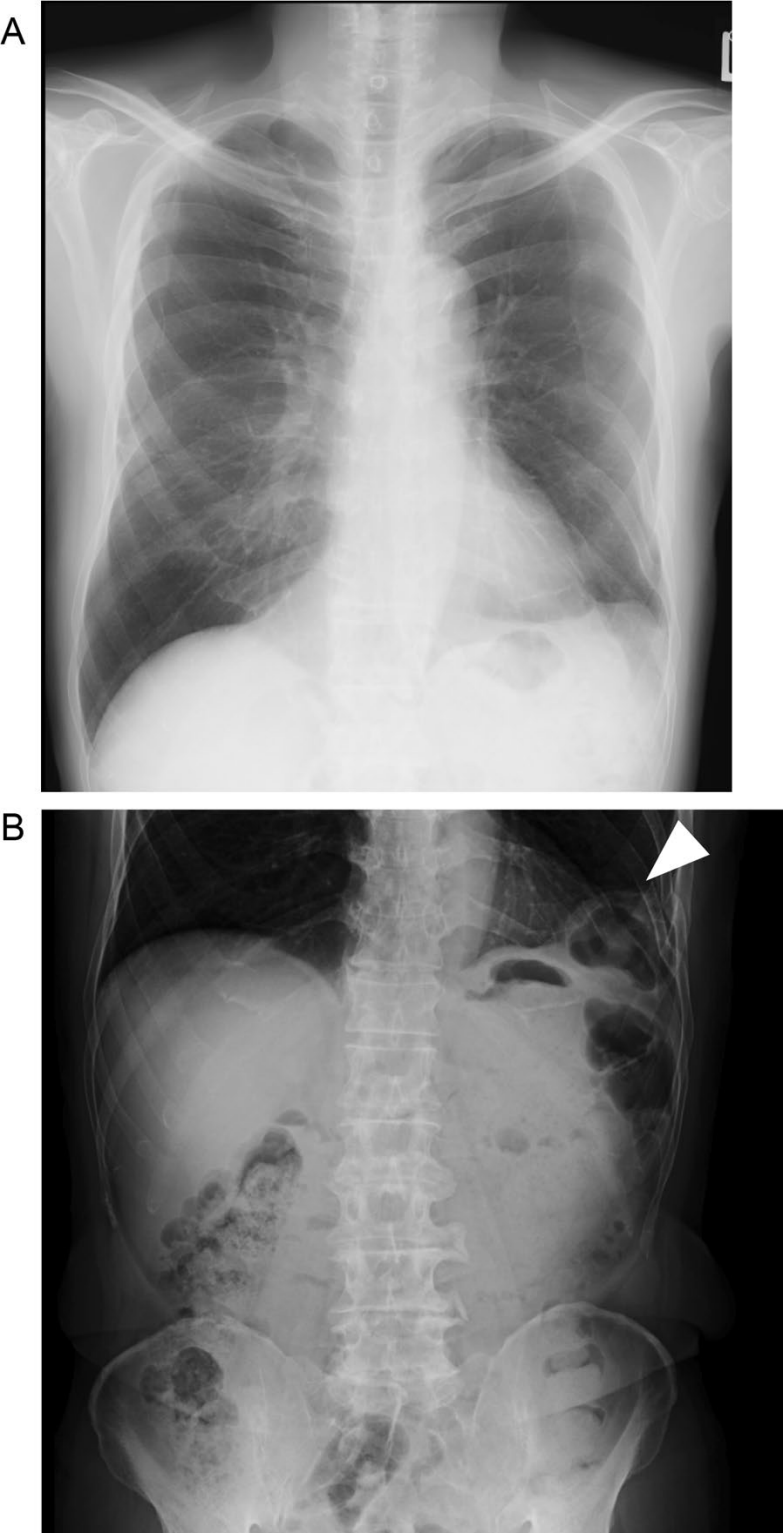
Preoperative radiographs. Chest radiographs show intra-abdominal tissue evacuated into the left thoracic cavity in the absence of pain (A) and presence of pain (B). We confirmed the colon incarceration (white arrowhead)
